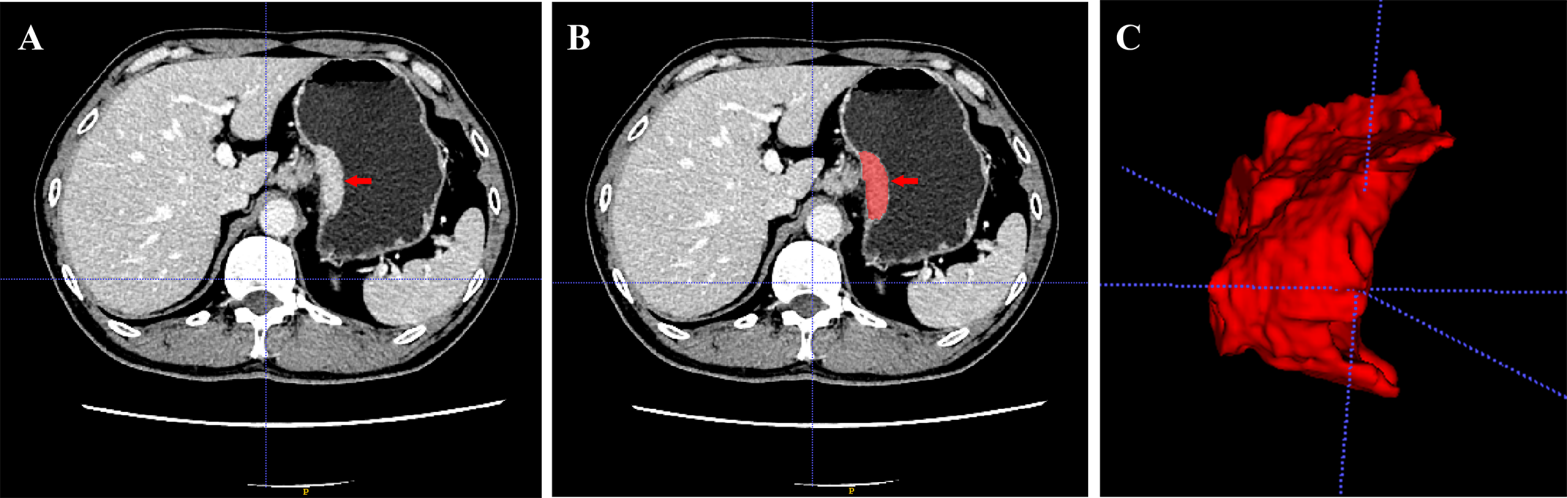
Manual segmentation of tumor with a GC patient. (A) A slice portal venous phase of contrast-enhanced CT images of the tumor. (B) the red label masks a slice CT image of the tumor with manual segmentation. (C) Three-dimensional (3D) image of the tumor with manual segmentation.
